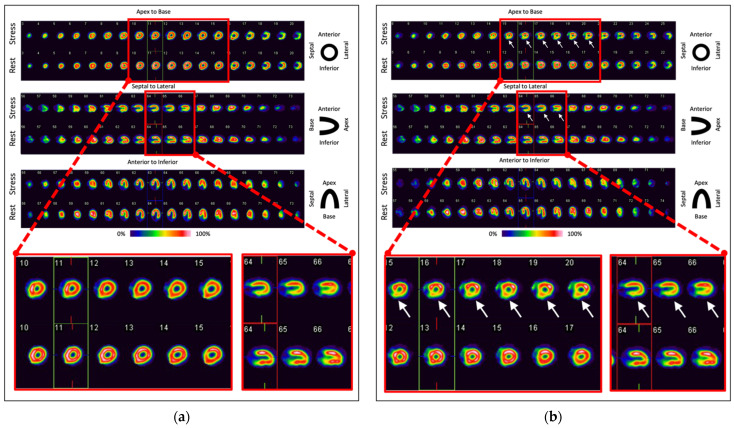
(a) Example for a physiological result in myocardial perfusion SPECT: No stress-induced perfusion defects, no ischemia, normal LVEF in stress and rest conditions (≥55%). (b) Example for a pathologic result: Stress-induced perfusion defects of the posterior wall (white arrows, ischemia: 10% of left-ventricular myocardium), normal LVEF in stress and rest conditions (≥55%). Images were acquired at our institution.
